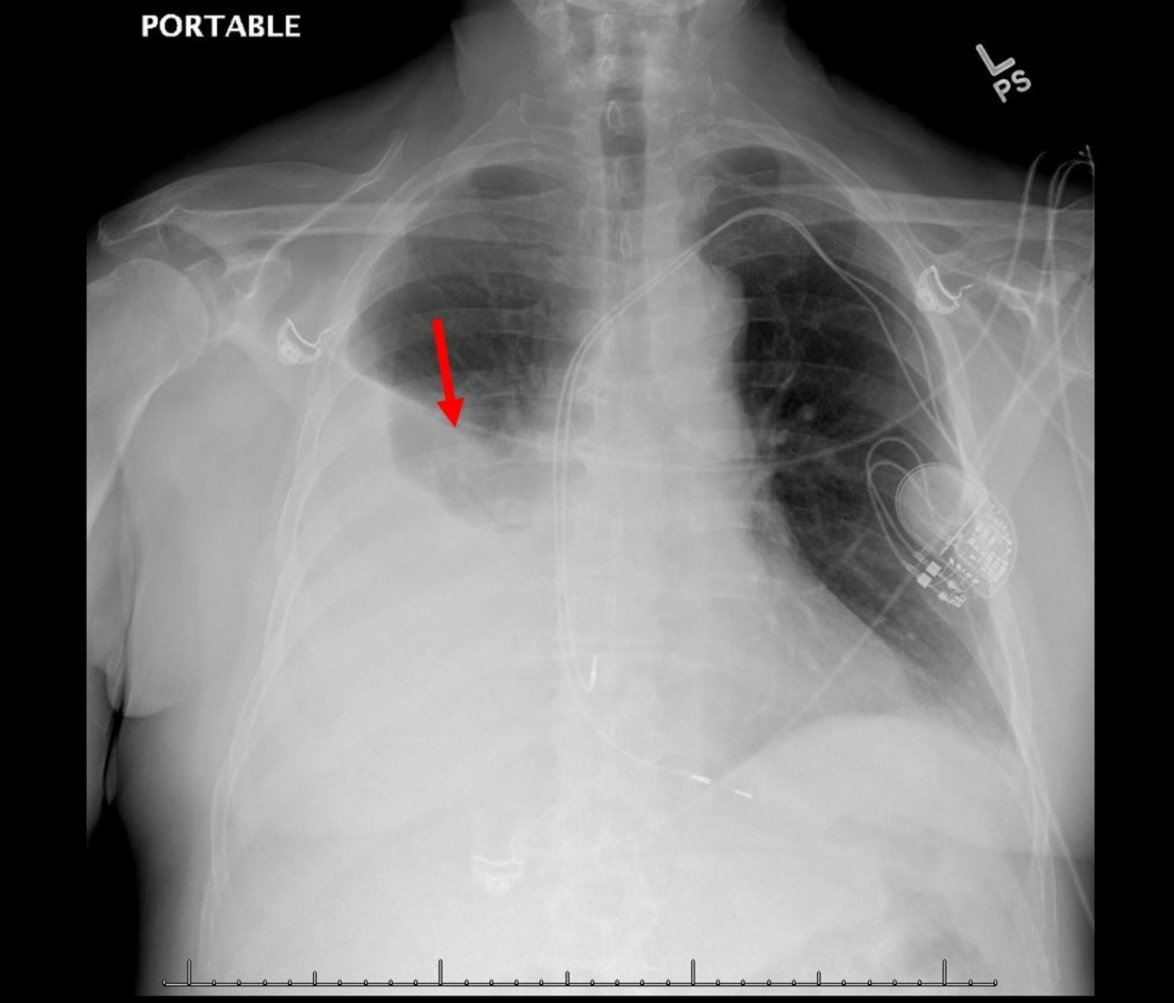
Chest radiograph (anterioposterior view) revealing large right-sided pleural effusion (red arrow)
